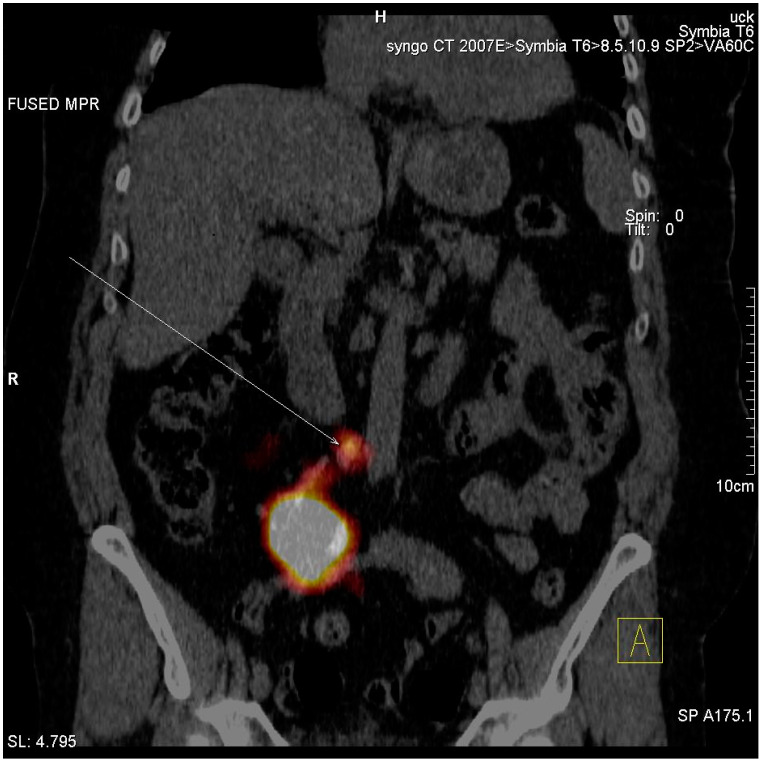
SPECT/CT example of lymphatic outflow from the right renal pelvis caudally and medially to the paraaortic region.
